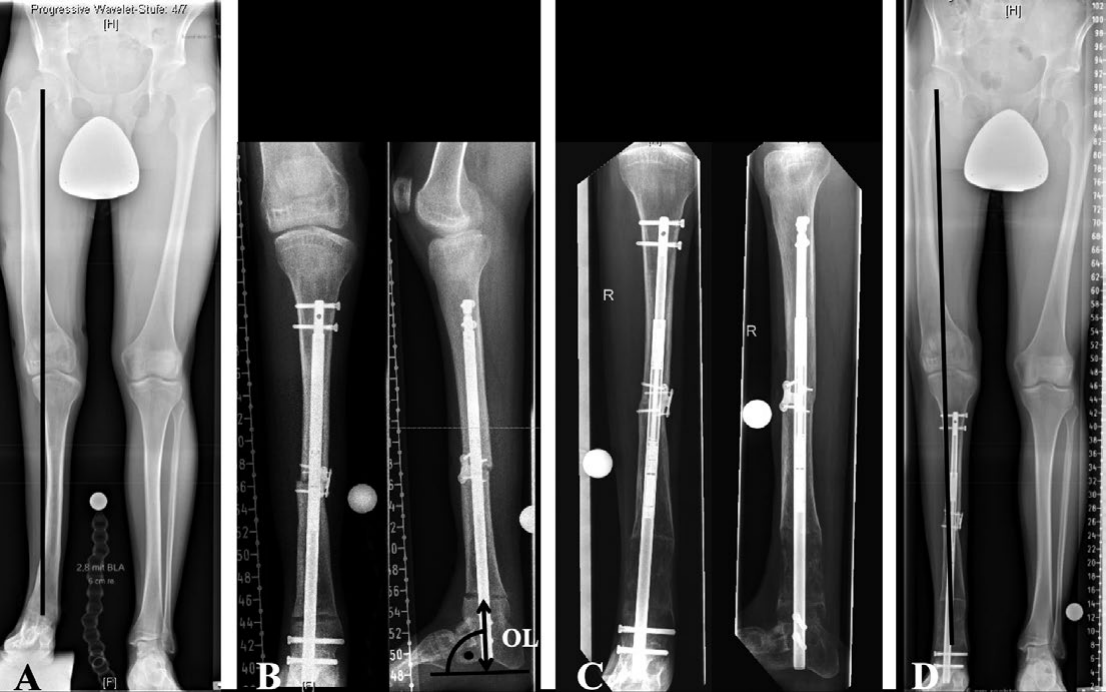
Patient No. 9 with additional osteotomy for angular correction. A—Preoperative radiographs showing LLD of 75 mm and mid-shaft tibial valgus malalignment. B—A second osteotomy was performed on the apex of the deformity for angular correction in addition to a distal corticotomy for callus distraction. The osteotomy level (OL) is measured as the distance from the tibial osteotomy site (drawn as a line parallel to the nail) to a perpendicular line aligned tangentially with the most plantar aspect of the calcaneus on the lateral radiograph (70.4 mm, meta-diaphyseal). The mid-shaft osteotomy was bridged by the ILN and additionally fixed using a locking plate to prevent proximal distraction. C—Full consolidation of the mid-shaft osteotomy and beginning callus consolidation after distraction of 65 mm. D—Postoperative radiograph showing a residual LLD of 11 mm and correction of the tibial valgus malalignment.
